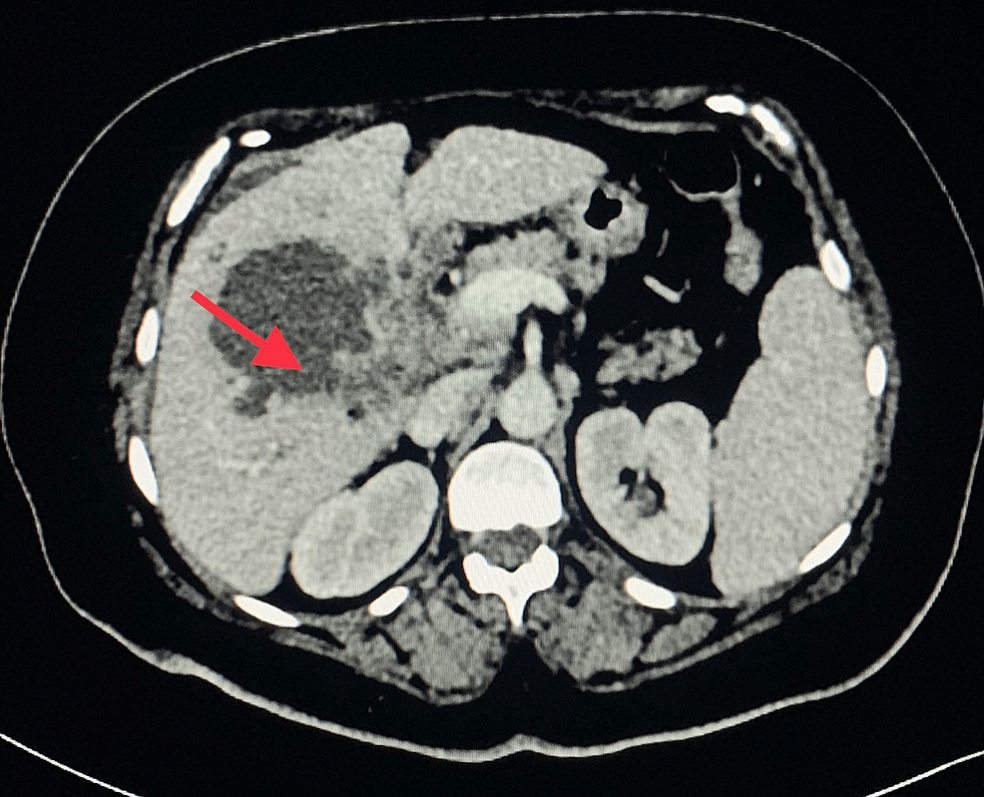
CT axial view showing the rent in the GB wall with a 6 × 7 cm hepatic abscess in liver segment IV. CT: computerized tomography, GB: gallbladder.
